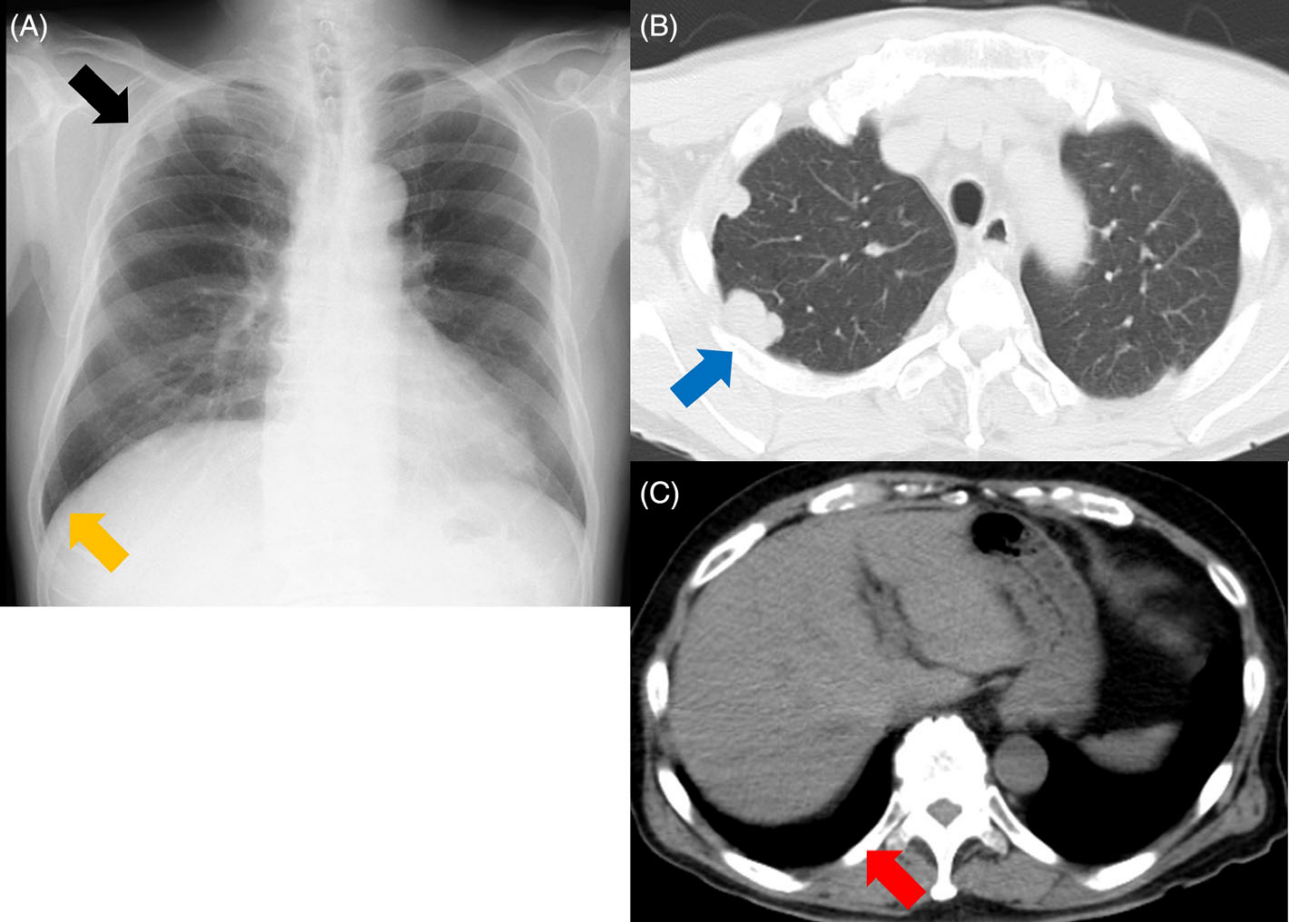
Chest x‐ray showed an abnormal shadow in the right upper pulmonary lung field (black arrow) but did not show pleural effusion (yellow arrow) (A). Chest computed tomography showed a nodular shadow bordering the pleura (blue arrow) (B) but did not show pleural effusion (red arrow) (C).
